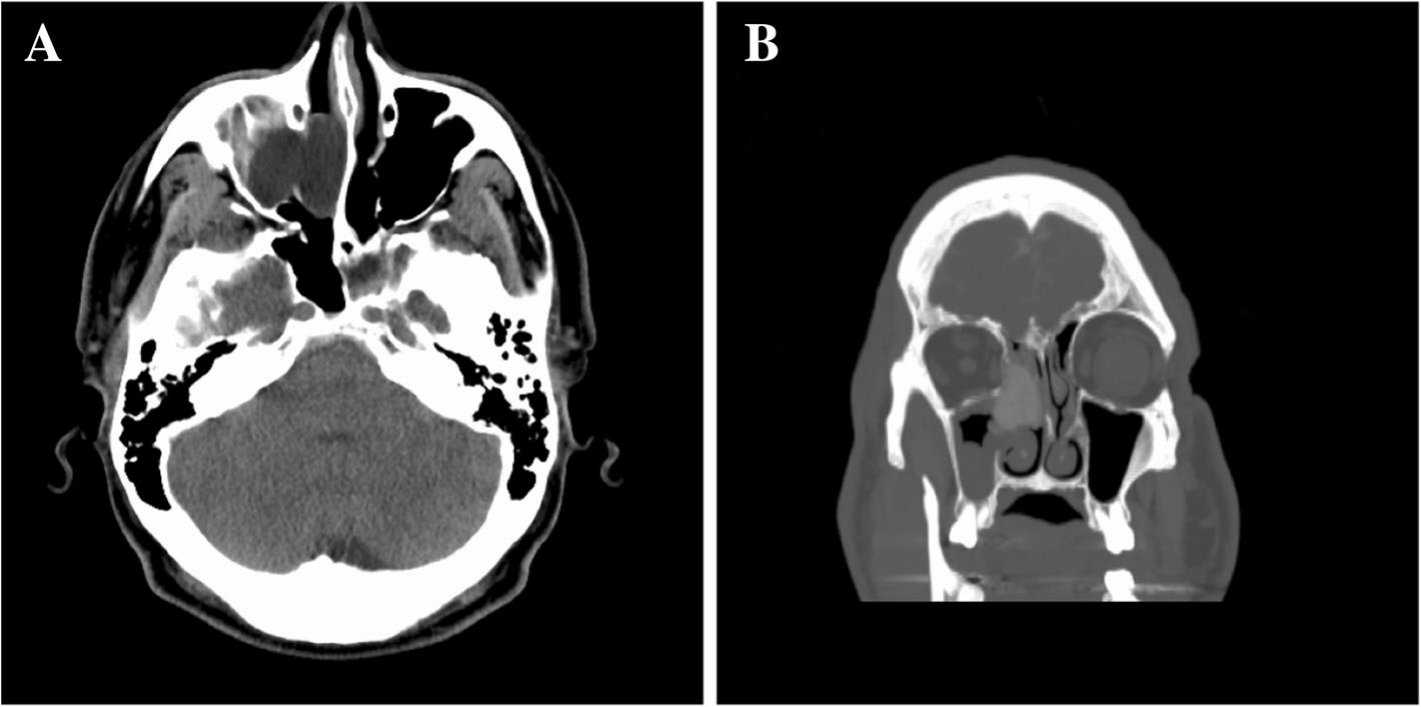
Computed tomography without contrast, preoperative images. a Axial and b coronal computed tomography images showed bony defects in the left lateral sphenoid sinus and right anterior cribriform plate
